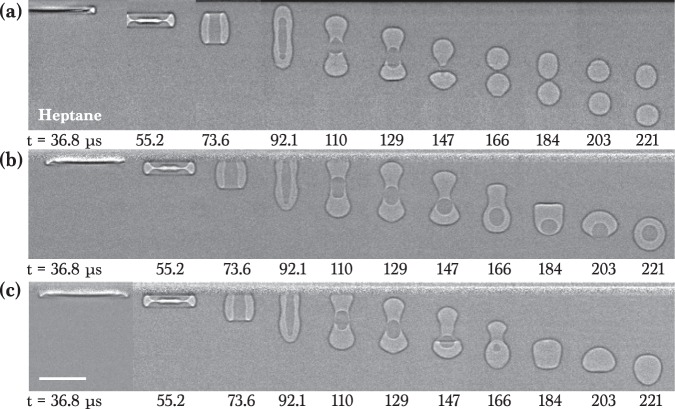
Sequential X-ray images of evolution of the entrapped bubble for the heptane. (a) The axisymmetric coalescence of the daughter droplet and the surrounding liquid splits the bubble into a double bubble. (b) No coalescence results in an antibubble comprising the daughter droplet inside. (c) One-sided coalescence of the daughter droplet with the surrounding liquid results in one bubble. The scale bar is 100 *μ*m long.
